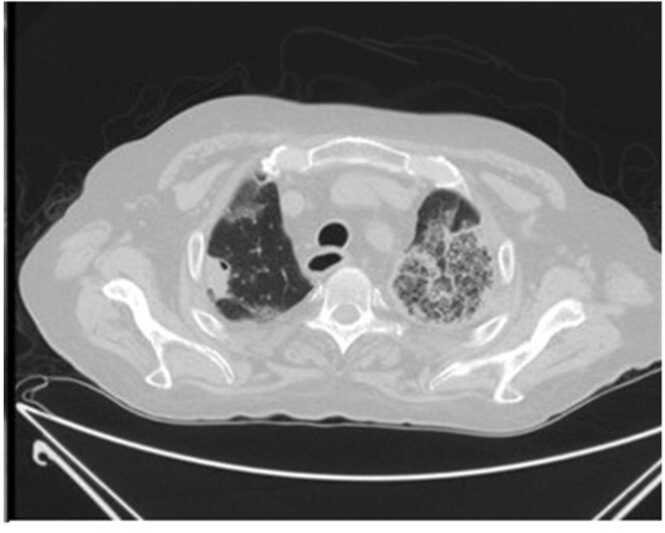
Chest CT scan showing bilateral ground glass opacities and consolidations in lower lobes of the lungs.
